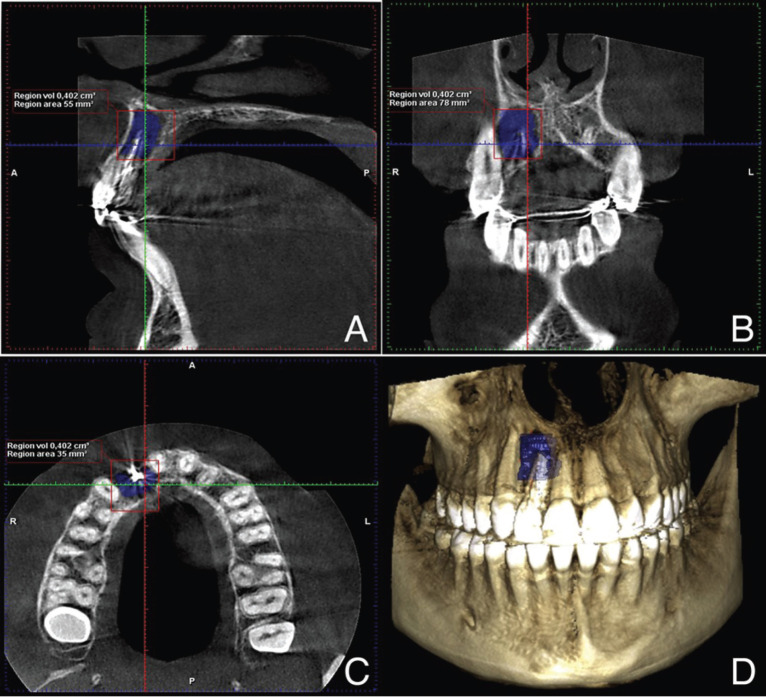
Volumetric assessment of a periapical lesion of a lateral upper right incisor using CBCT. A) Coronal plane. B) Sagittal plane. C) Axial view. D) 3D image of the traced lesion.
